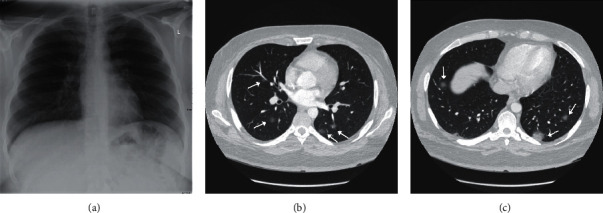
A 26-year-old male with a recent diagnosis of diabetes presented with several days of progressive myalgia, cough, chills, and dyspnea and intermittent generalized crampy mild abdominal pain. (a) The chest radiograph was negative for consolidation. (b, c) The CT pulmonary arteriogram was performed for the same patient to rule out pulmonary embolism and showed multiple patchy, lower-lobe-prominent, nodular ground-glass opacities (arrows). The patient was tested positive for COVID-19.
